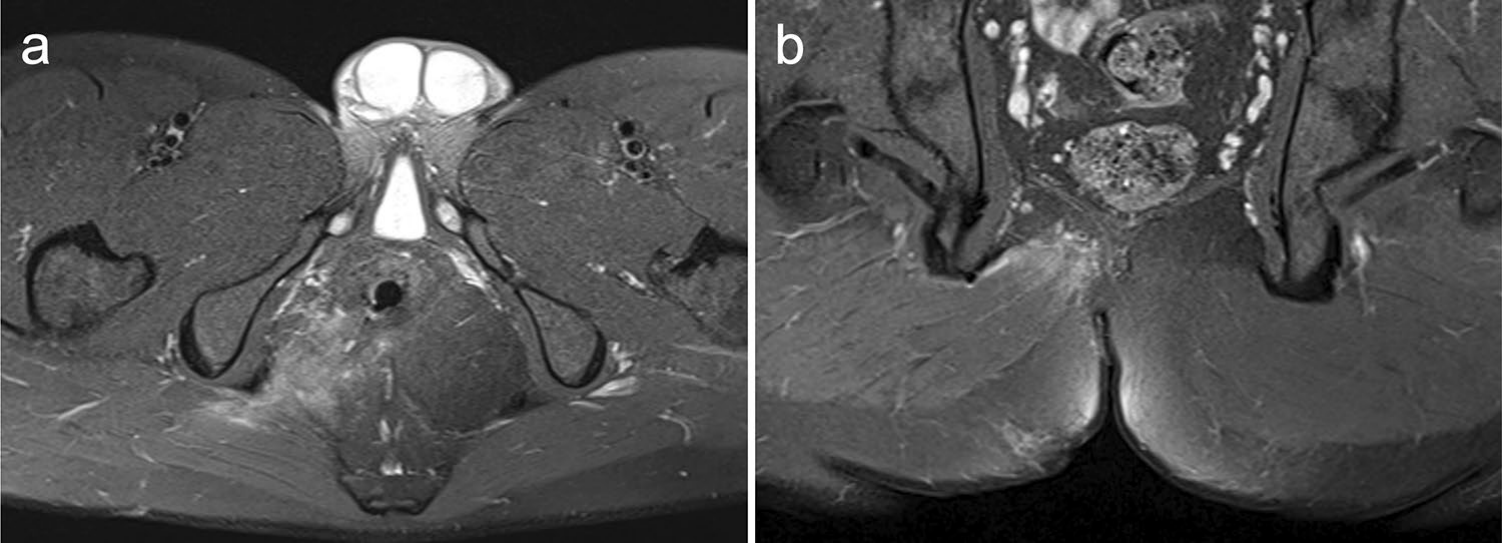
MRI 5 months after the operation. Imaging showed excellent healing
